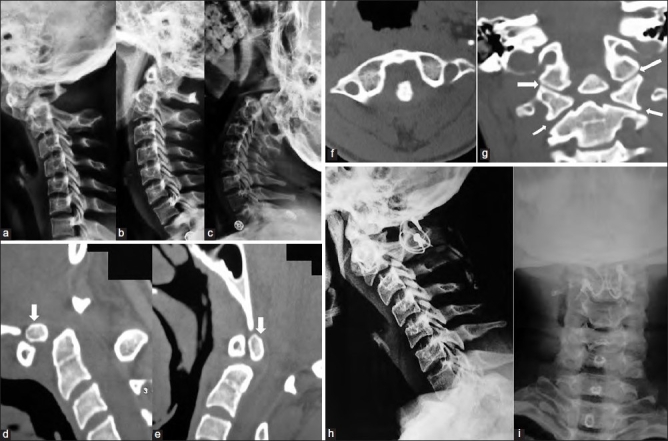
Lateral cervical spine radiograph showing AAD on neck flexion (a). C1 posterior arch is anterior to spinolaminar line and causing canal compromise. OO is impinging into the canal in neutral (b) and extended (c) neck positions. Sagittal CT in flexion (d)/ extension (e) showing anterior C1 arch-os complex (arrow) being above the tip of remnant odontoid and having anteroposterior translational movements unhindered by the latter. Axial CT (f) showing AAD. Coronal CT (g) showing OO and symmetrical facet joints (arrows). Lateral radiograph of CVJ (h) showing posterior sublaminar C1-2 fusion using so’fwires. OO and the remnant odontoid-C2 body are in alignment. C1-posterior arch and C2 lamina are closely approximated. Anteroposterior radiograph (i) shows orientation of bilaterally placed sof’wires
